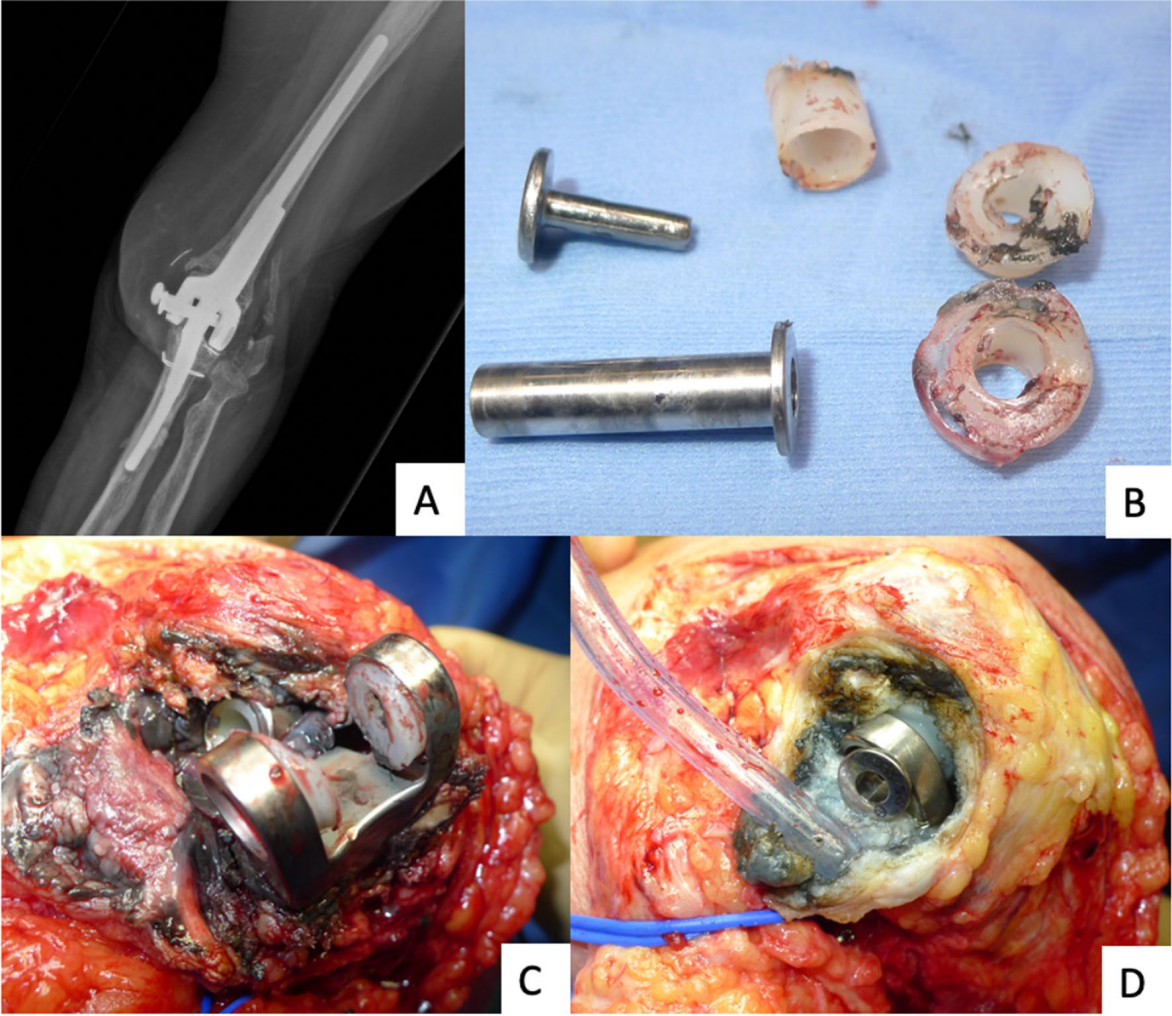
(A) X-ray of pin loosening after wearing of bushing’s polyethylene component; (B) (C) (D) intraoperative images of pin loosening after asymmetrical wearing of bushing’s polyethylene component
